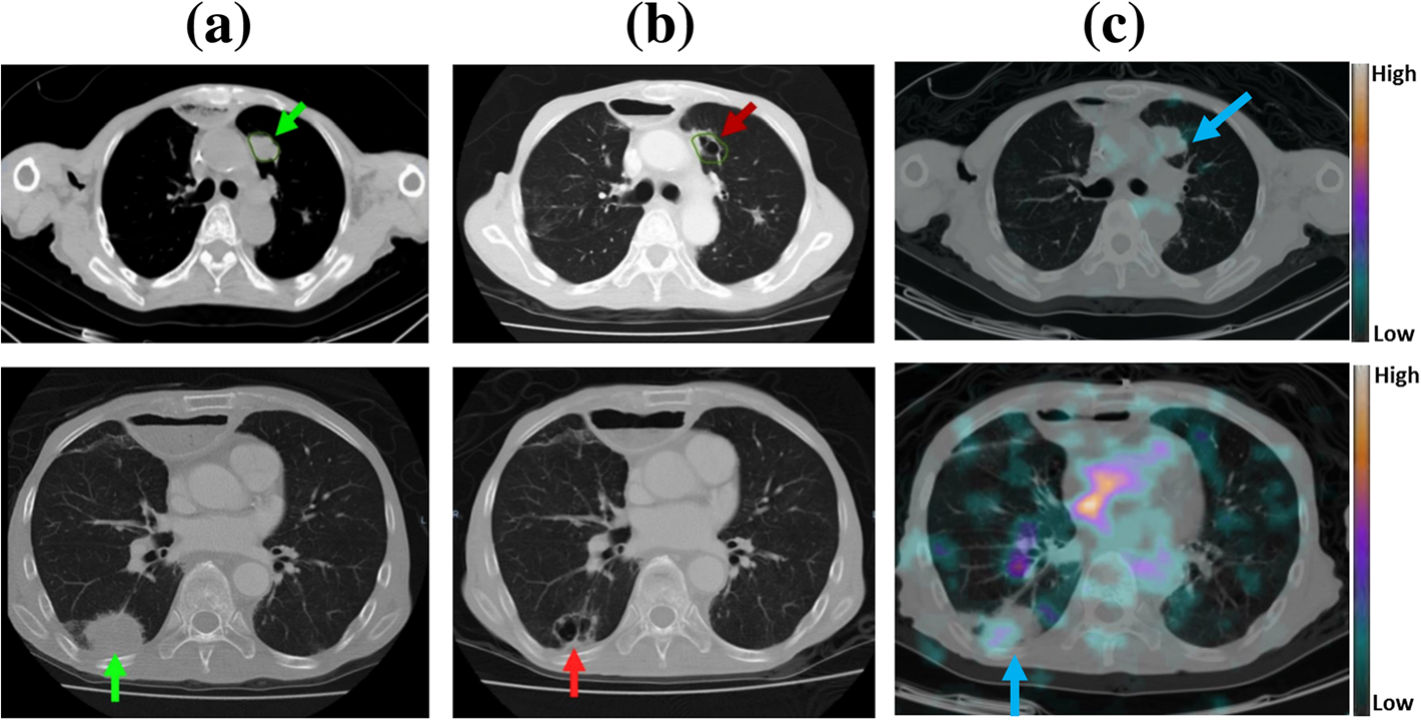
A series of chest CT images for patient 11, who had esophageal cancer and lung metastases tumors: a 1 month before and b 4 months after ^188^Re-liposome injection, the metastatic lesions (green arrows) either decrease in size or show signs of cavitation (red arrows) and c SPECT/CT images captured at 8 h after ^188^Re-liposome injection show a high uptake and efficient targeting in the corresponding tumor lesion (blue arrow), which is seen on CT
